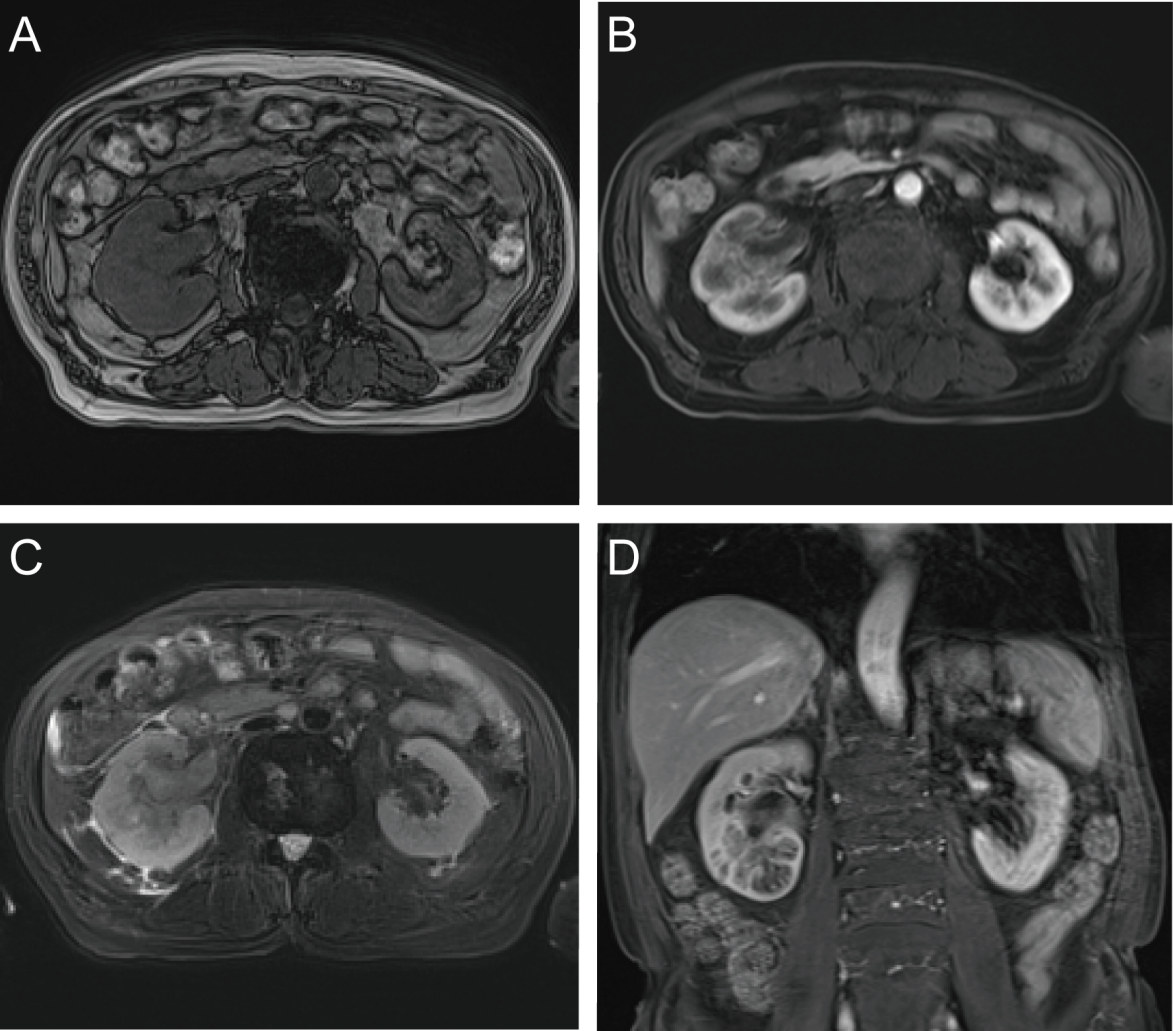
Enhanced MRI examination. (A) axial T1-weighted imging; (B) axial T1-weighted arterial phase imaging; (C) axial T2-weighted imging; (D) coronal T1-weighted arterial phase imaging.
